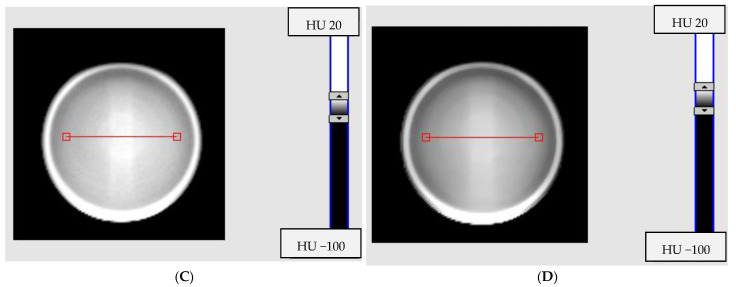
Two-dimensional images (a transversal plane, Z = −1 mm) of irradiated PABIG^nx^ as measured with CBCT and iCBCT (TrueBeam accelerator, Varian). The dosimeter in the PH-6-DD2 container (GeVero Co.): (A) is for the CBCT scan, 1 series, (B) is for the iCBCT scan, 1 series, (C) is for the CBCT with a mean of 5 series and (D) is for the iCBCT with a mean of 5 series. Pelvis mode was used for scanning. The red lines in (A)−(D) indicate the position of profiles presented in Figure 2.
